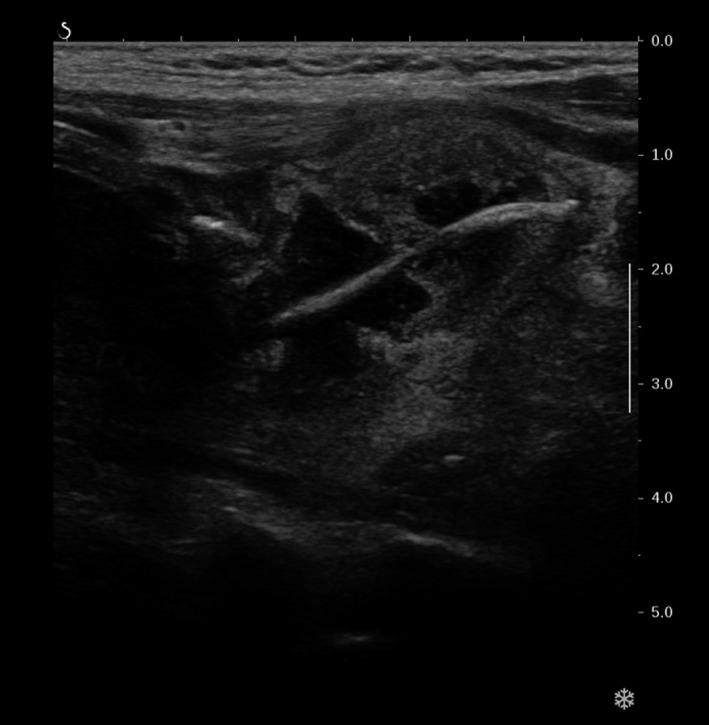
Ultrasonography image showing the fish bone in the left lobe of the thyroid gland surrounded by an abscess
